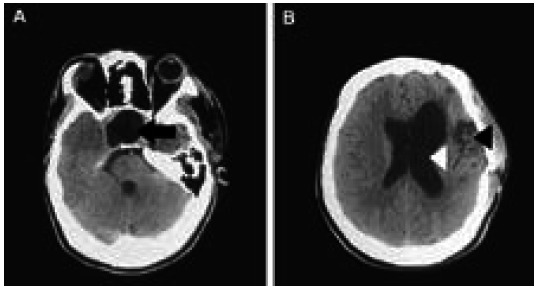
Postoperative CT scan of the brain.
